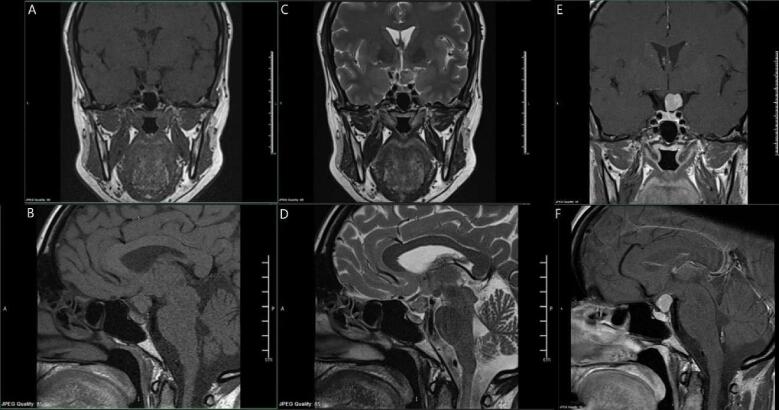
Preoperative MRI, A & B are T1 MRI, C&D are T2 MRI, E & F are T1 MRI with contrast, showing A well-defined completely suprasellar homogenously enhancing mass lesion is seen arising from the left lateral aspect of the pituitary infundibulum abutting the left optic tract and tuber cinereum measuring approximately 1.1 × 1.2 cm. Pituitary gland is seen separately. The lesion shows intermediate signal intensity on T1 and T2-weighted images.
